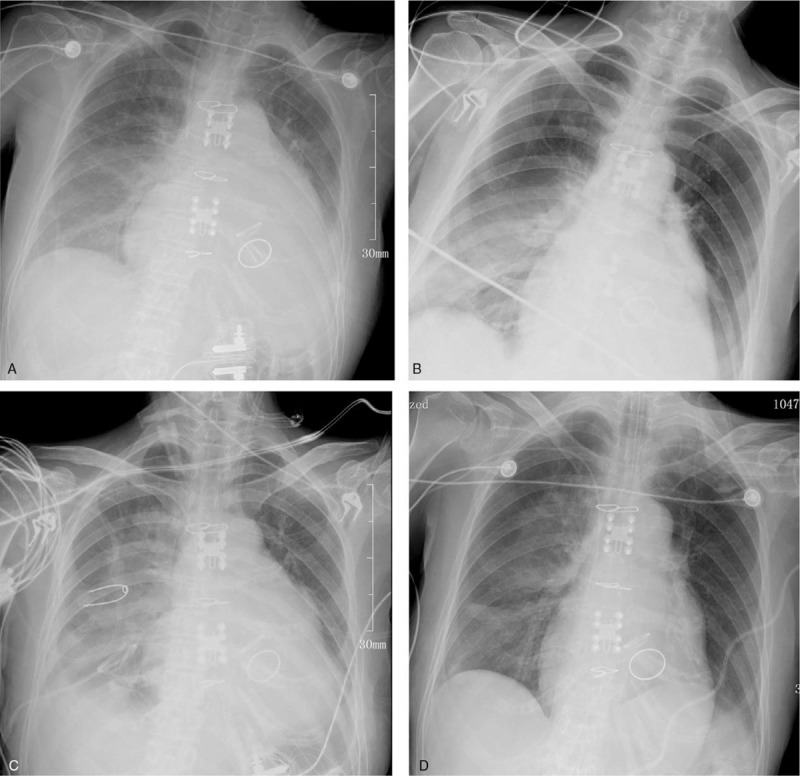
Dynamic changes in chest X-ray during hospitalization. (A). On February 16, the chest X-ray showed that the lung was unclear. (B). On February 22, the chest X-ray presented the consolidation in the right lower lung. (C, D). Following polymyxin B intravenously administered, the lung consolidation dissipated and clear.
